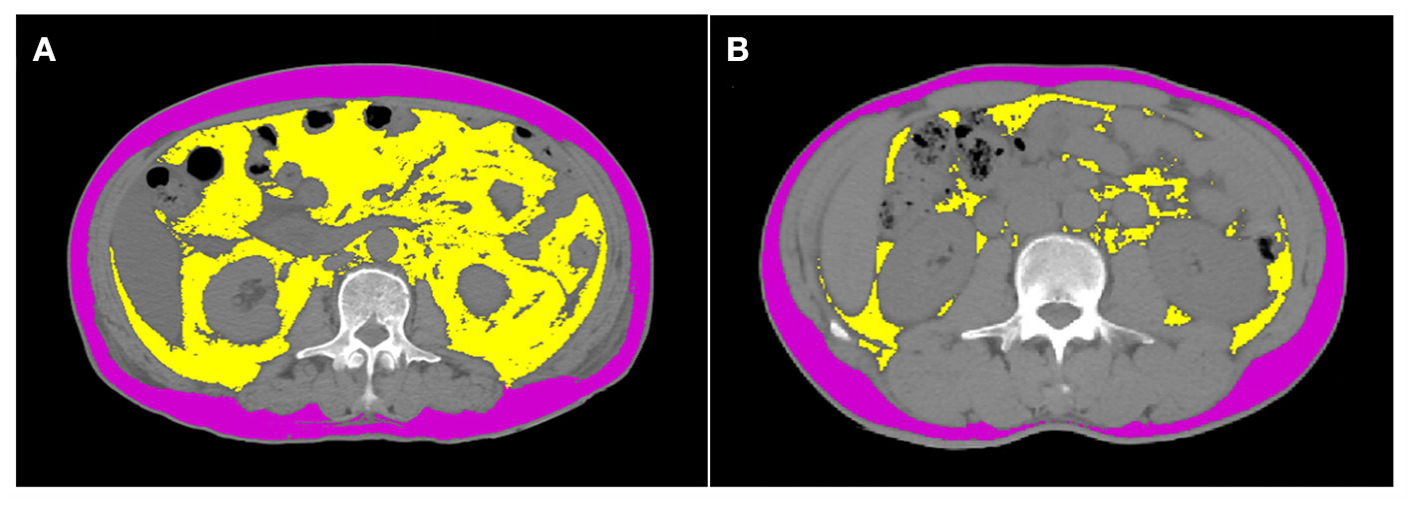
Computed tomograph scan shows the body composition of normal BMI individuals with and without visceral obesity. (A) represents a normal BMI with visceral obesity, with a BMI of 23.2 kg/m^2^ and visceral adipose tissue of 207 cm^2^. (B) represents a normal BMI without visceral obesity, with a BMI of 23.2 kg/m^2^ and visceral adipose tissue of 23.9 cm^2^. Visceral adipose tissue area is highlighted in yellow and the subcutaneous adipose tissue area is purple.
